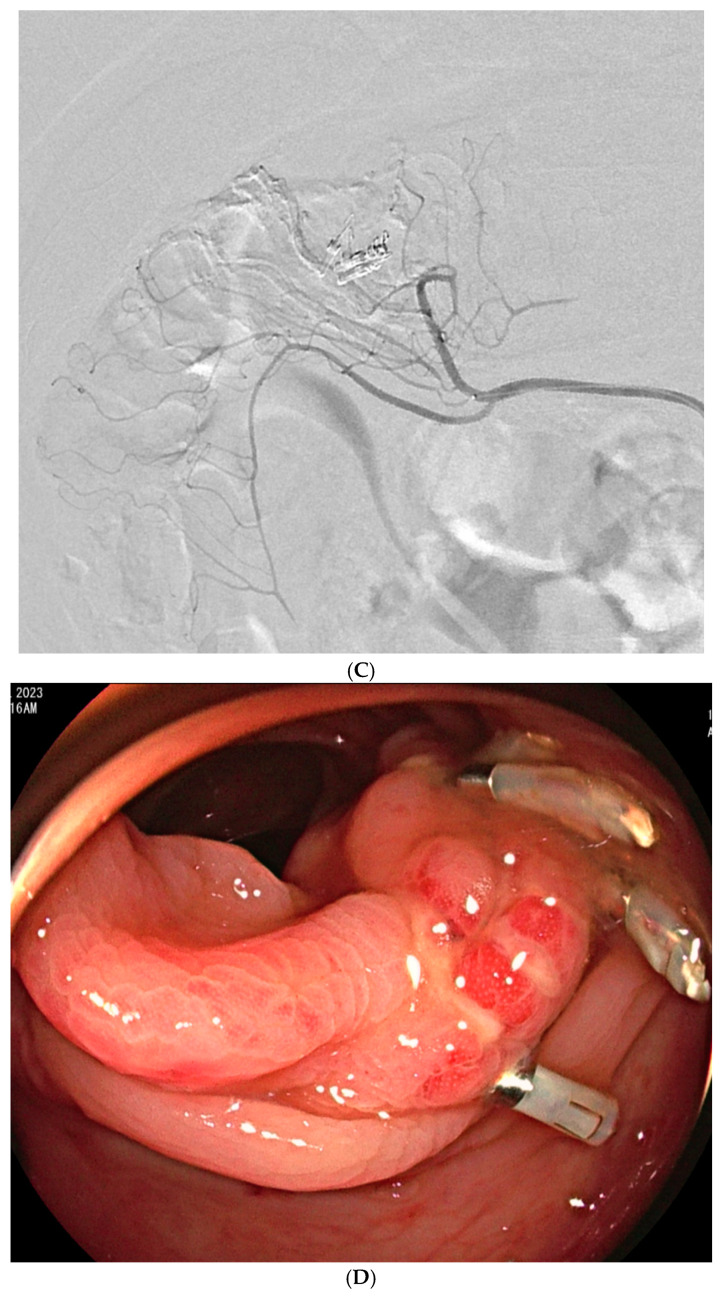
A 59-year-old man with colonic diverticula presented with hematochezia. (A) Contrast-enhanced axial CT image shows colonic diverticula with luminal contrast extravasation (arrow) at the hepatic flexure. Sigmoidoscopy performed one day after the CT scan reveals a diverticulum with bleeding (inset). Endoscopic hemoclipping was performed. (B) Superior mesenteric arteriography demonstrates focal hypervascularity (arrows) around the endoclipping site, without evidence of contrast extravasation. As superselection was not possible, the arterial branches leading to the endoclipping site were embolized using quick-soluble gelatin sponge particles (150–350 µm). (C) Post-embolization arteriography shows marked reduction in the previously observed hypervascularity around the endoclipping site. (D) Sigmoidoscopy performed two days after embolization reveals several ulcers and erythematous changes around the hemoclipping site, consistent with ischemic colitis. Associated abdominal pain resolved within three days. No further bleeding occurred during the 6-month follow-up.
